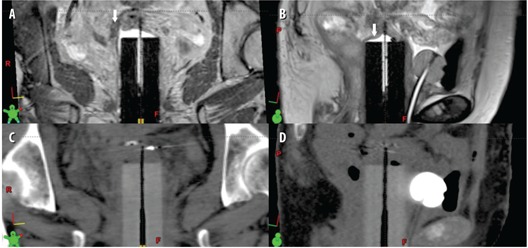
Paracoronal ((a) and (c)) and parasagittal ((b) and (d)) T2‐weighted MR and CT, respectively, through the custom interstitial applicator.
